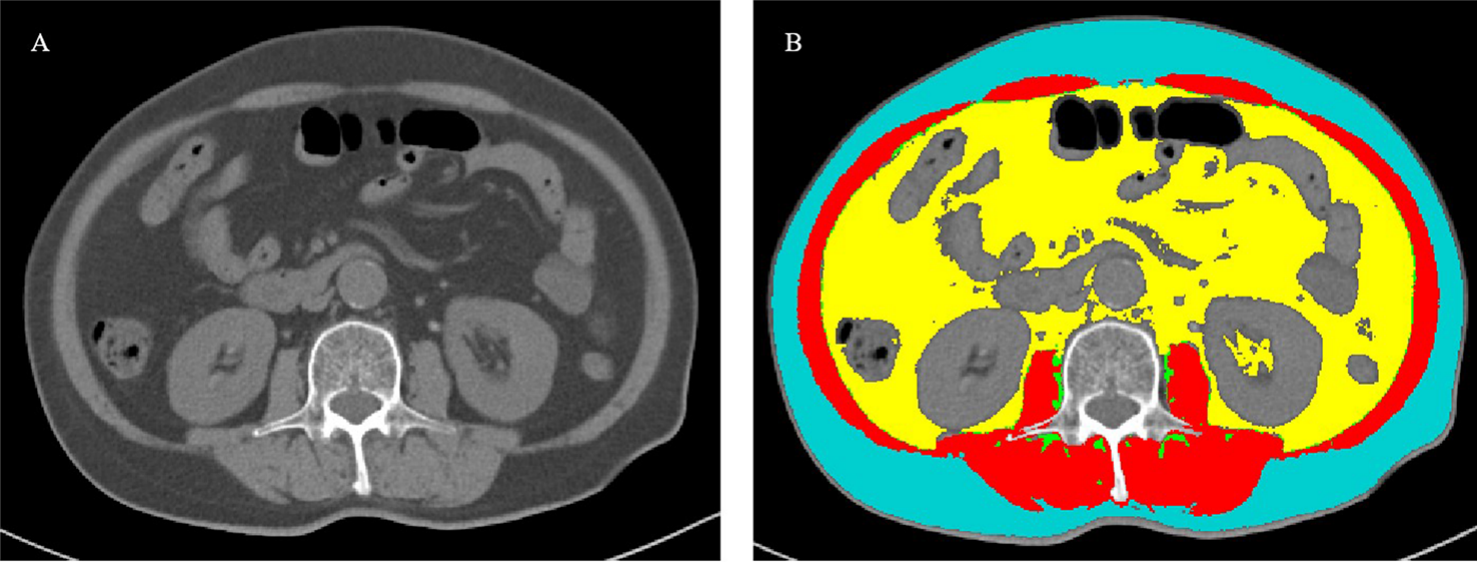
Measurement of body composition parameters with cross-sectional CT images at the third lumbar level. (A) A cross-sectional CT image at the third lumbar level. (B) Body composition automatically calculated using the ABACS Auto Segmentation module in SliceOmatic software (ver. 5.0). Visceral adipose tissue, subcutaneous adipose tissue, intramuscular adipose tissue, and muscle were targeted as yellow, blue, green, and red, respectively.
